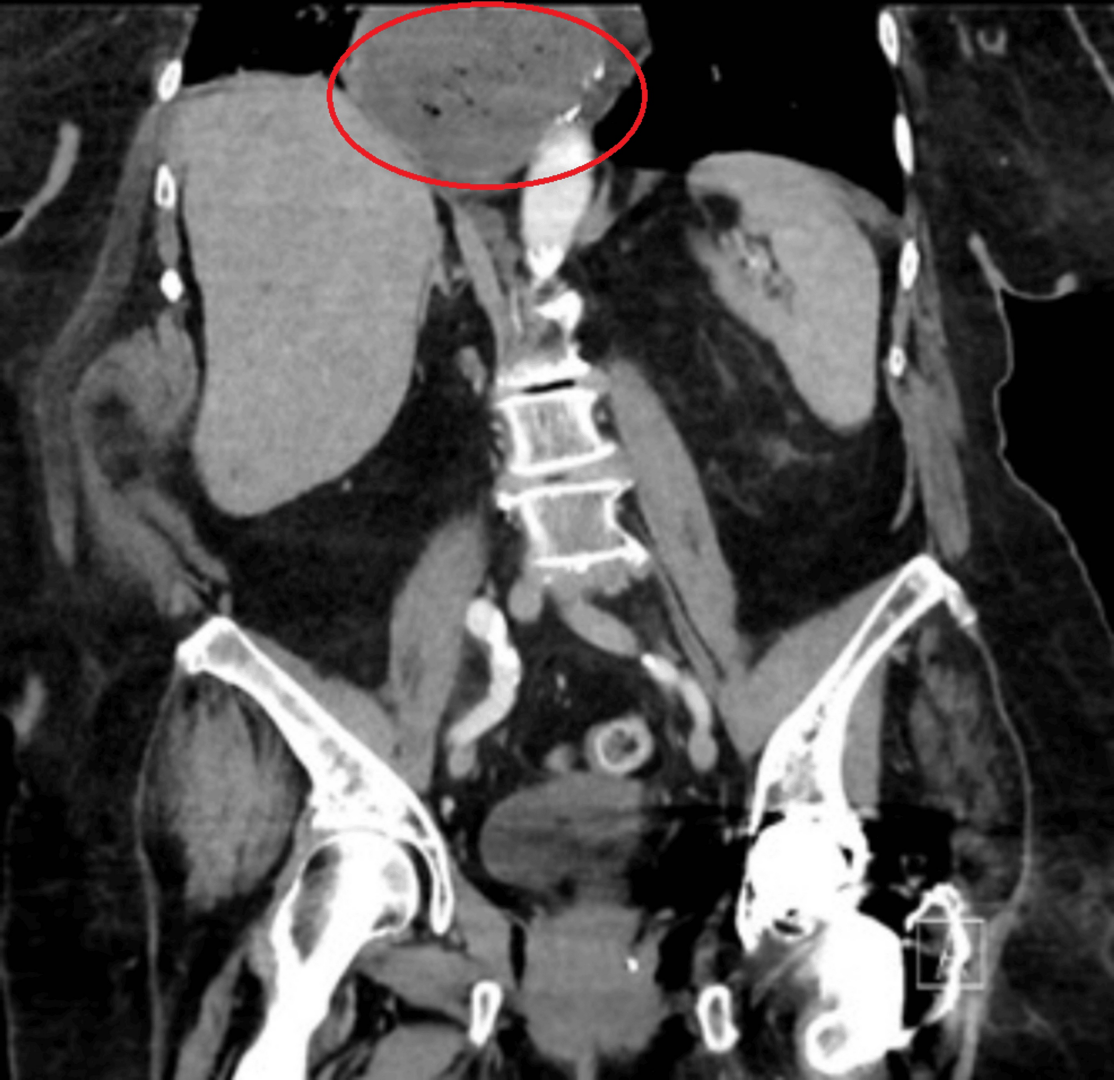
CT abdomen and pelvis demonstrating gastric sleeve herniated into the mediastinum Red circle indicates the gastric sleeve within the hiatal hernia.
